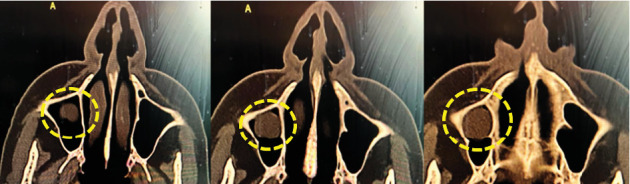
CT scan showing a well-defined, dome-shaped retention cyst in the right maxillary sinus. The cyst is exerting light pressure against the sinus walls, likely correlating with the patient's reported symptoms of extreme discomfort.
